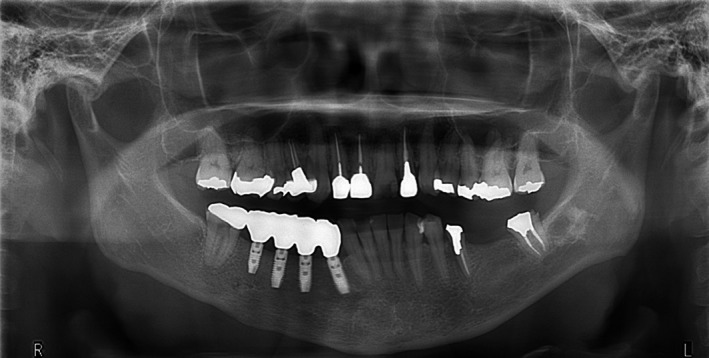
Orthopanoramic radiograph obtained 5 y after the operation
